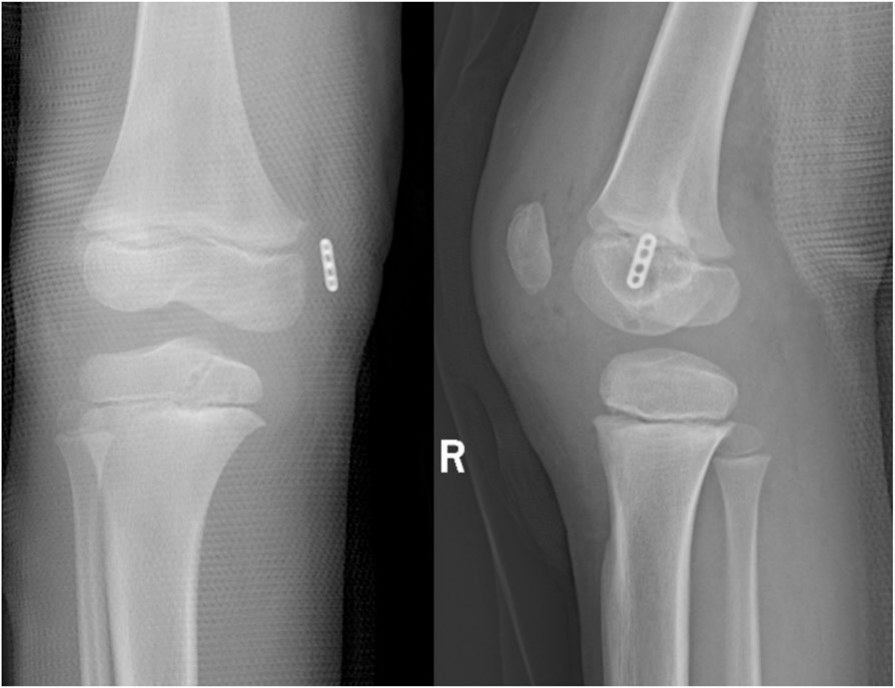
Post-operation X-ray. The cortex-fixation button was placed distal to the growth plate; the gap between the button and cortex is the epiphyseal cartilage
